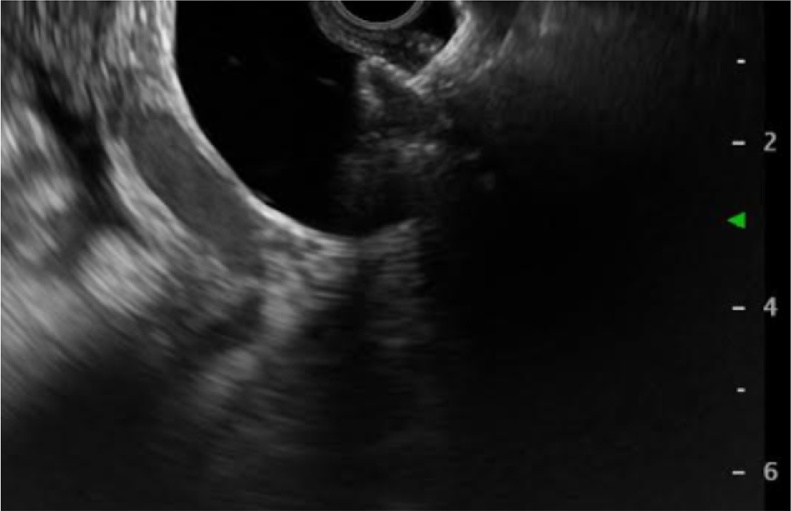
Endoscopic ultrasound vision shows the distal flange of LAMS (HOT AXIOS) in to the CBD.
